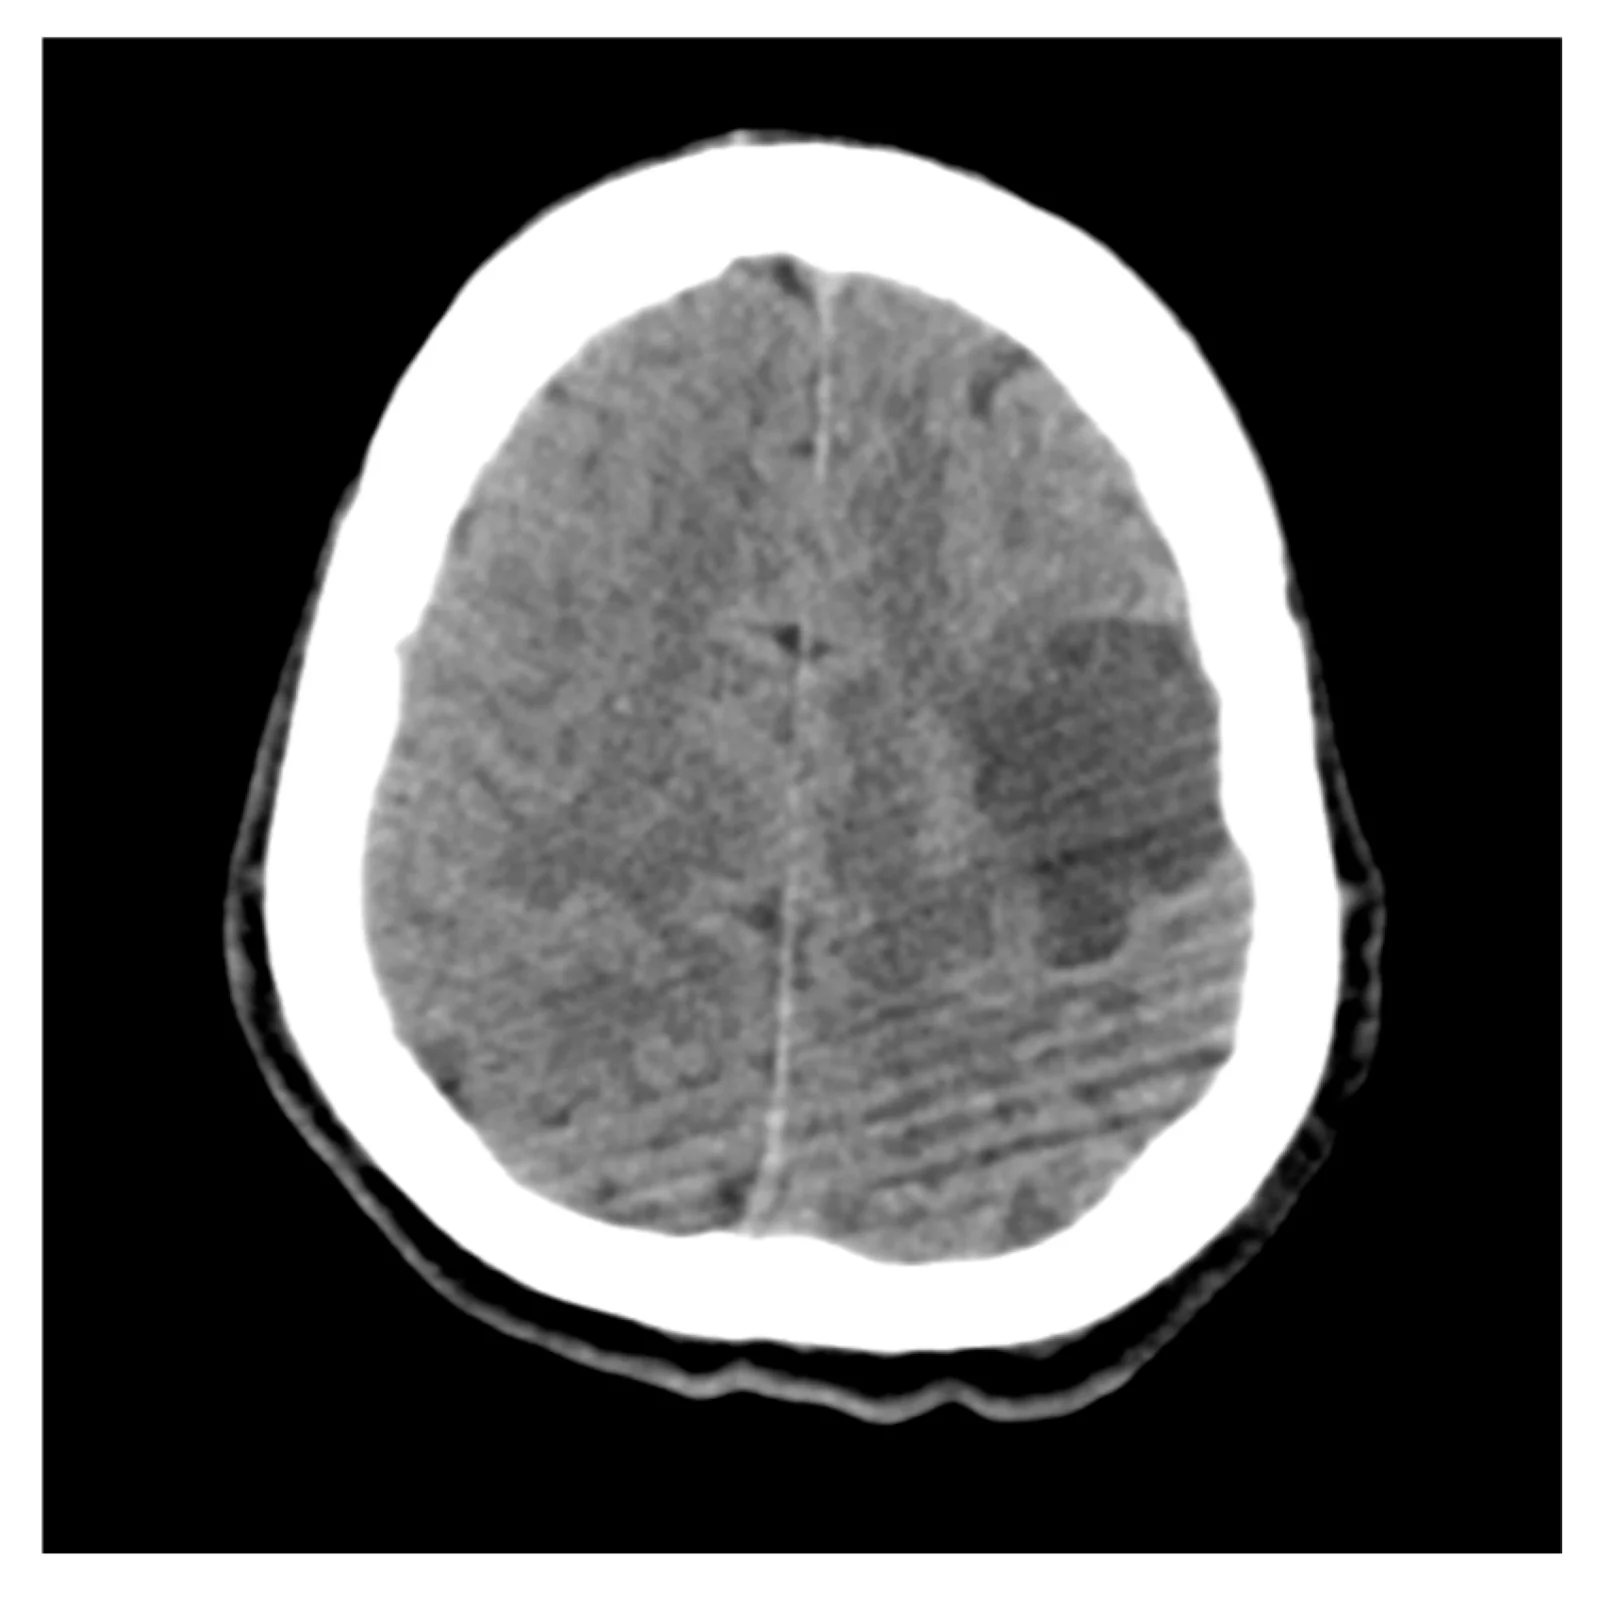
Follow-up CT imaging demonstrating new left frontoparietal ischemia.
